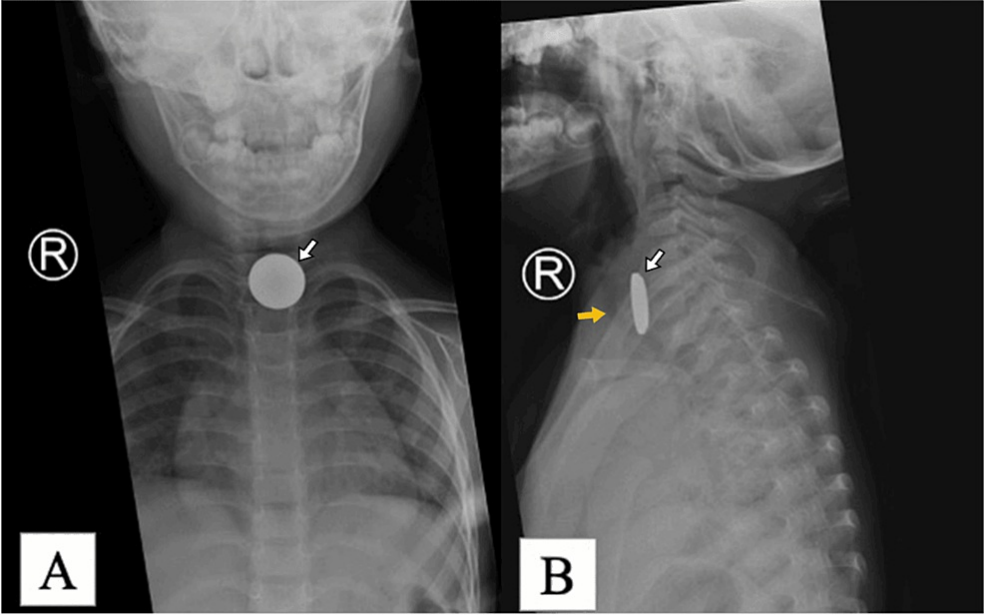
The cervical and chest X-ray (A) The anteroposterior (AP) view looks like a double ring shadow at closer view, while (B) the lateral view X-ray demonstrates the characteristic rounded edges and groove with step-off (arrow) radiopaque shadow foreign body. The view also revealed the position of foreign body in the oesophagus by located posteriorly to the trachea air shadow (yellow arrow).
